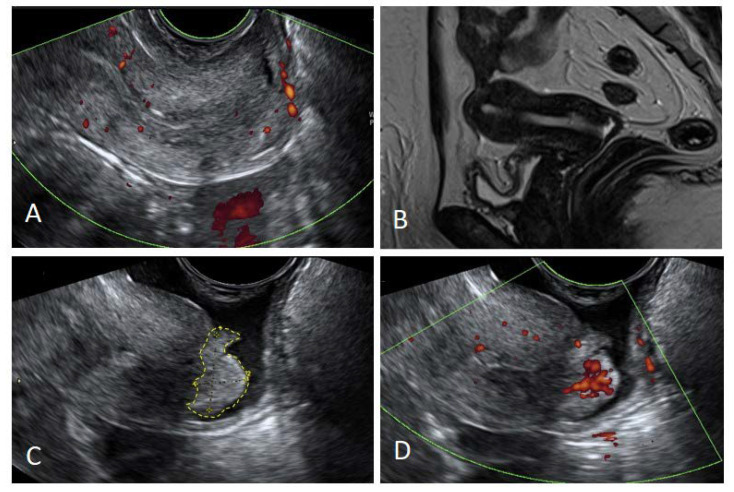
Detection of small tumors with VGS. (A,B) Small tumor not detectable at TVUS and MRI; (C,D) the same tumor detected by adding VGS and PD.
